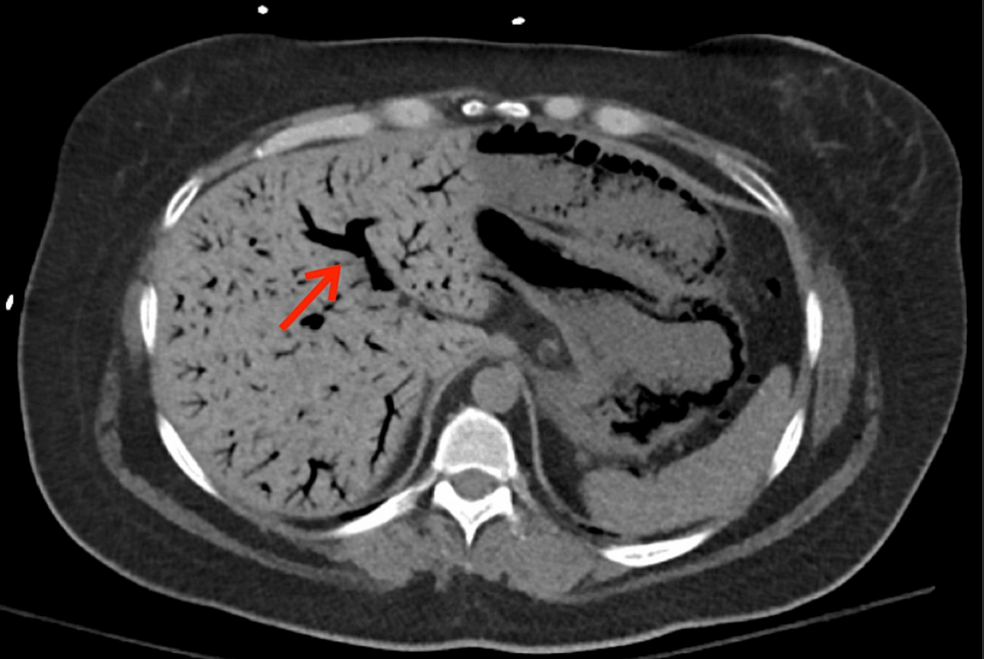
CT Scan of the abdomen showing portal venous gas
